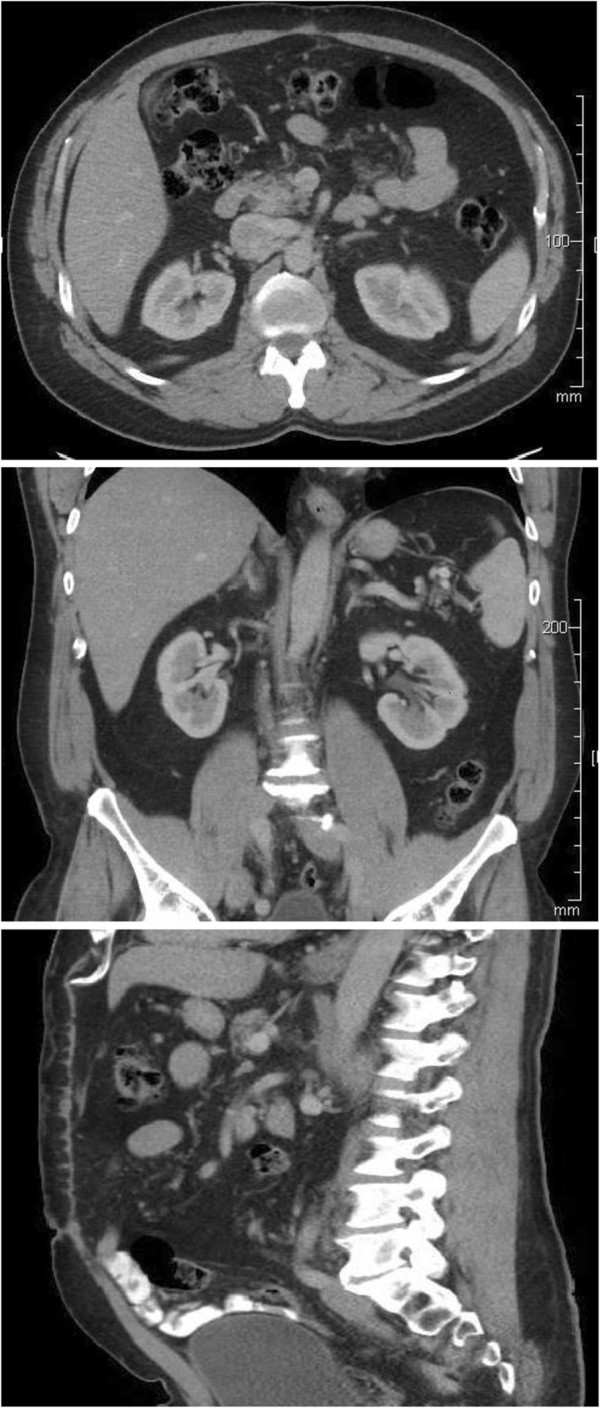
Post-operative computed tomography (CT) (axial, coronal and sagittal images).
